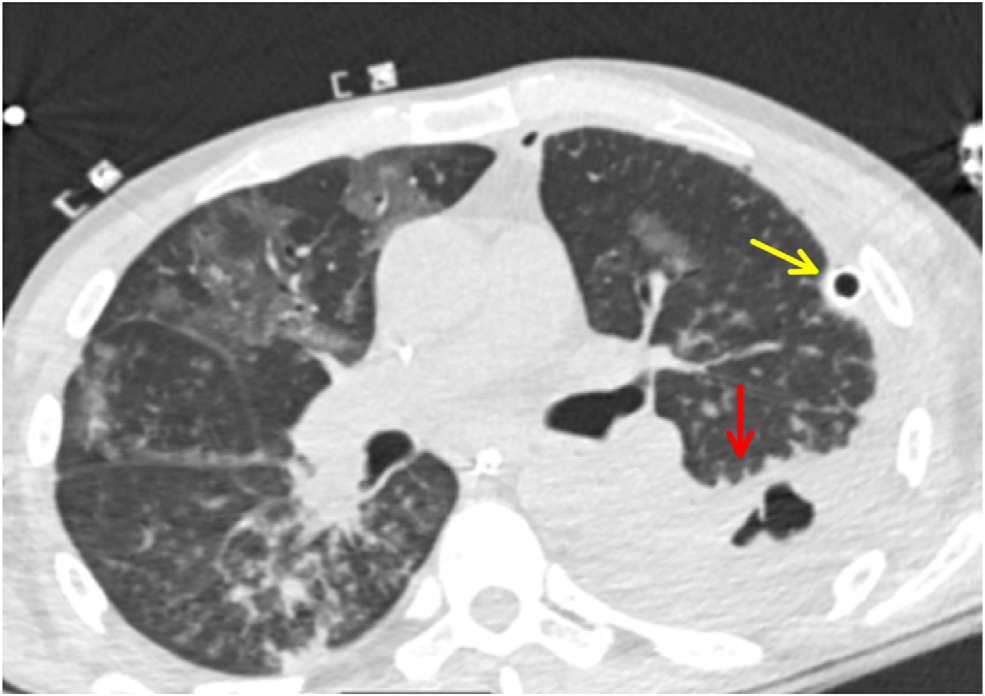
Repeat axial computed tomography chest with contrast on day six of intensive care unit admission showing persistent large empyema reduced in size (red arrow) with resolution of mediastinal shift. Chest tube still in place (yellow arrow), and bilateral ground-glass opacities present.
